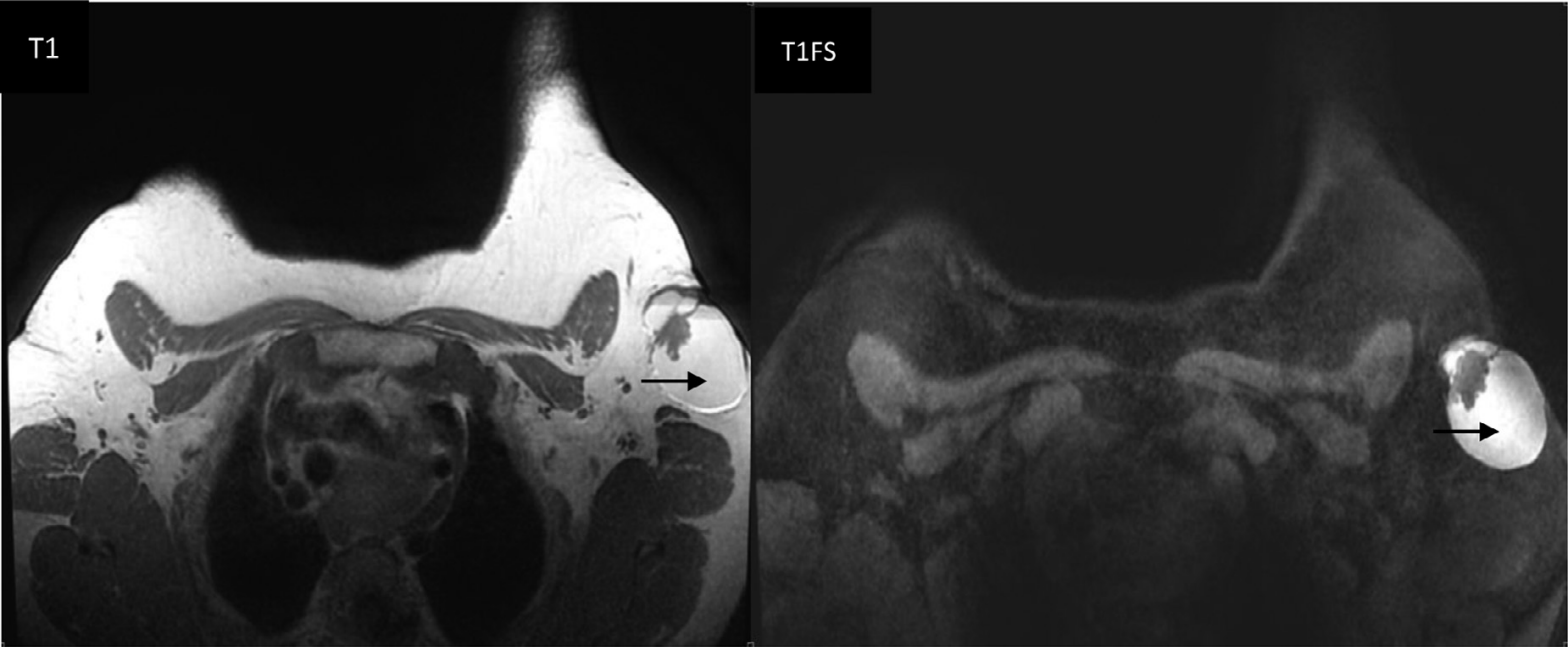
Axial T1-weighted image (left) and axial T1 with fat saturation sequence (right) showing the cystic mass in the left axilla with hemorrhagic content, that appears hyperintense in T1 not suppressed in fat saturation sequence (arrows).
